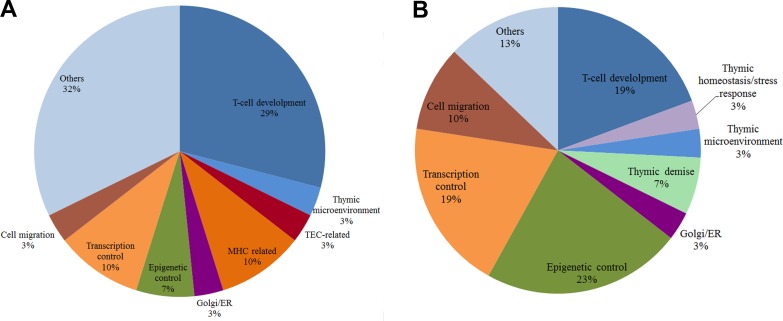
Pie chart of biological functions for high-hierarchy genes in CO networks Pie chart of HH gene functions in CT-CO network (A) and DS-CO network (B). Each slice represents the percentage of genes in a functional category.
